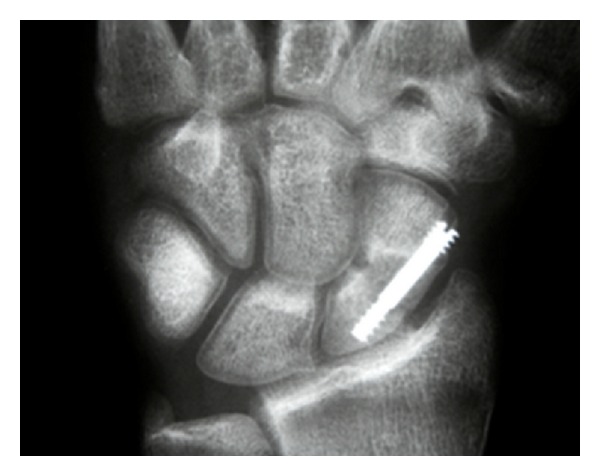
AP-radiograph of a 16-year-old patient in the follow up after screw fixation of a scaphoid fracture showing bandlike subchondral osteoporosis in all carpal bones.
